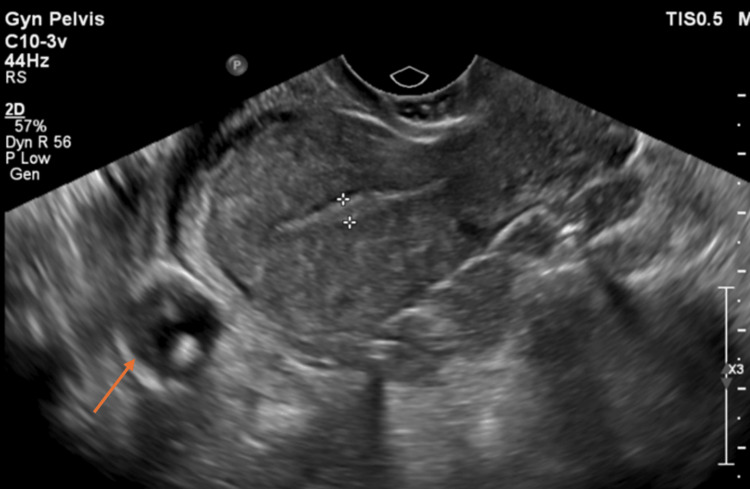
Transvaginal ultrasound showing an anterior abdominal wall cyst.
